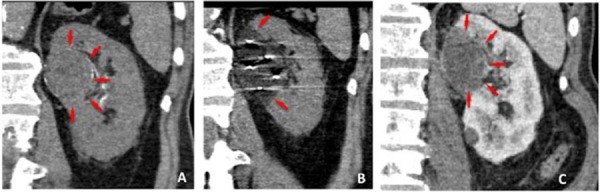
Coronal reformatted, CT of the left kidney (A) showing a central biopsy proven renal cell carcinoma (arrows). The intra-procedural coronal image shows the 5 cryyoprobes resulting in a lower density ice-ball (B, arrows). Three month follow up CT (C) shows complete lack of enhancement of the necrotic tumor (arrows).
